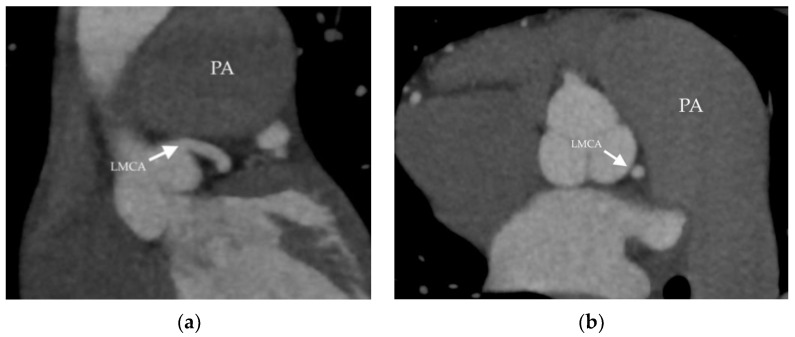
Coronary computed tomography angiography (Patient 2). The arrow points to the left main coronary artery (LMCA)- not compressed by the dilated pulmonary artery (PA): (a) sagittal view; (b) axial view.
